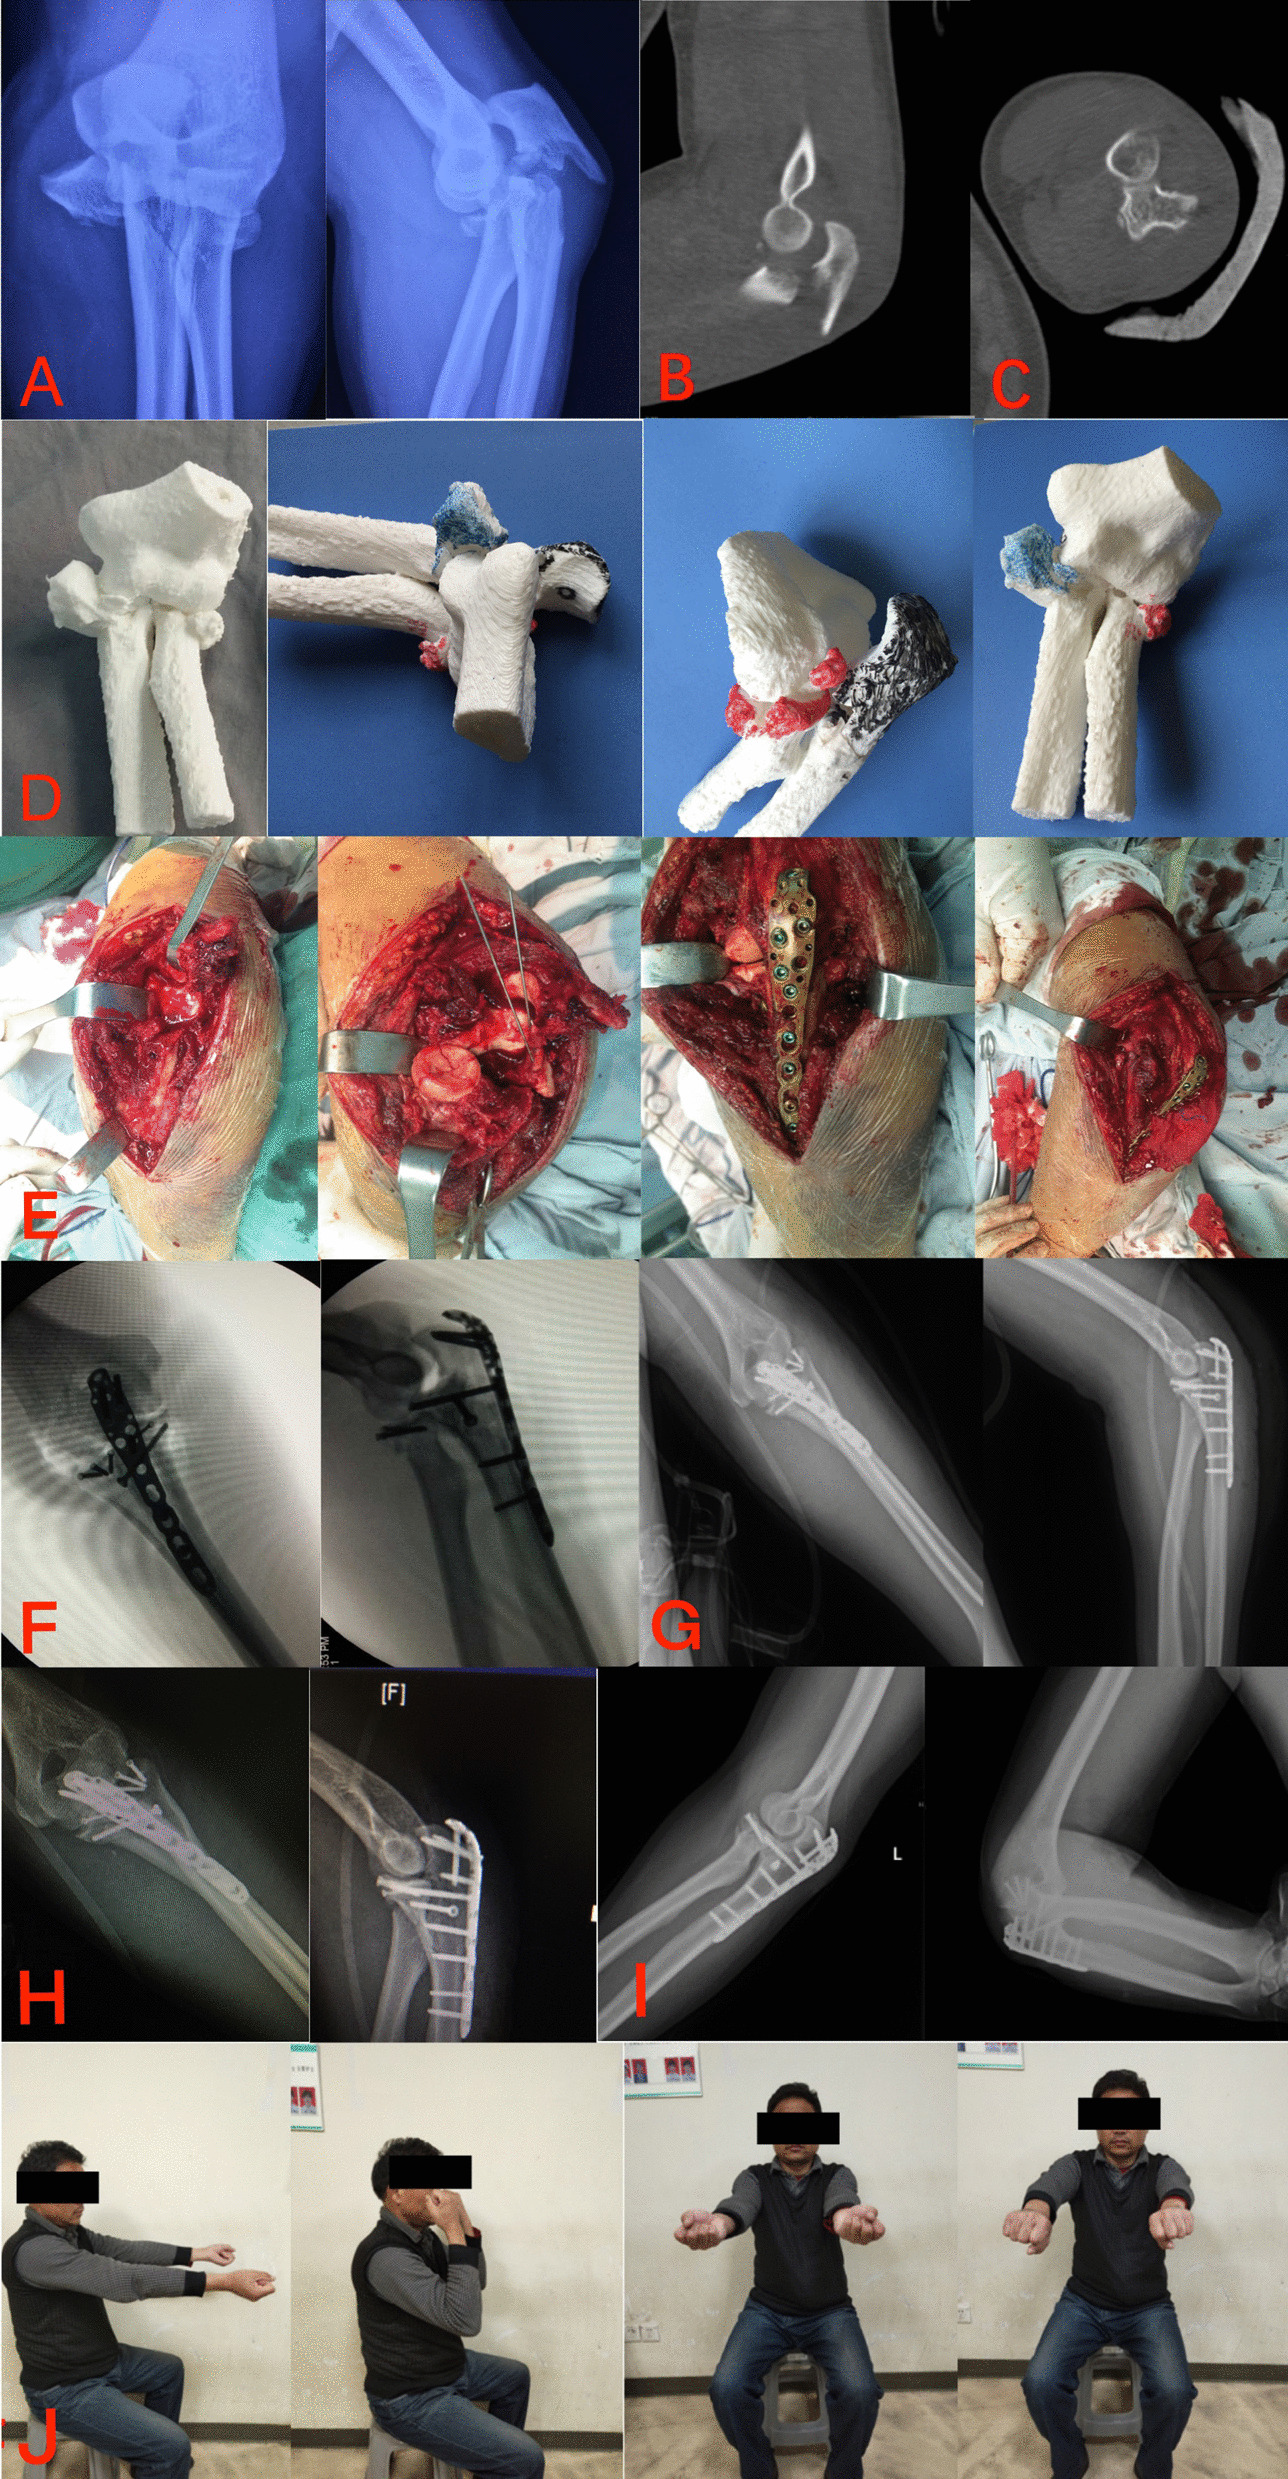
Male, 34 years old, falling caused left elbow injury. A Preoperative X-rays. B CT scan showed olecranon fracture and elbow posterior dislocation. C CT scan showed proximal radioulnar joint stable. D For better surgical guidance, we printed a 3D model, the olecranon fracture fragment is shown in black, the radial head fracture fragment in orange, and the coronoid process fracture fragment in blue. E Posterior midline approach was used, and the proximal olecranon fragment was identified and turned over. Coronoid process fragment and radial head fragment can be visualized due to the absence of olecranon obstruction. After the reduction and fixation of coronoid process and radius head, the olecranon fragments were also reduced and fixed. Finally, the lateral collateral ligament complex was examined and repaired if it was ruptured. F Intraoperative fluoroscopy. G Postoperative X-rays. H X-rays after surgery 6 months. I X-rays after surgery 1 year. J The functional assessment at last follow-up
